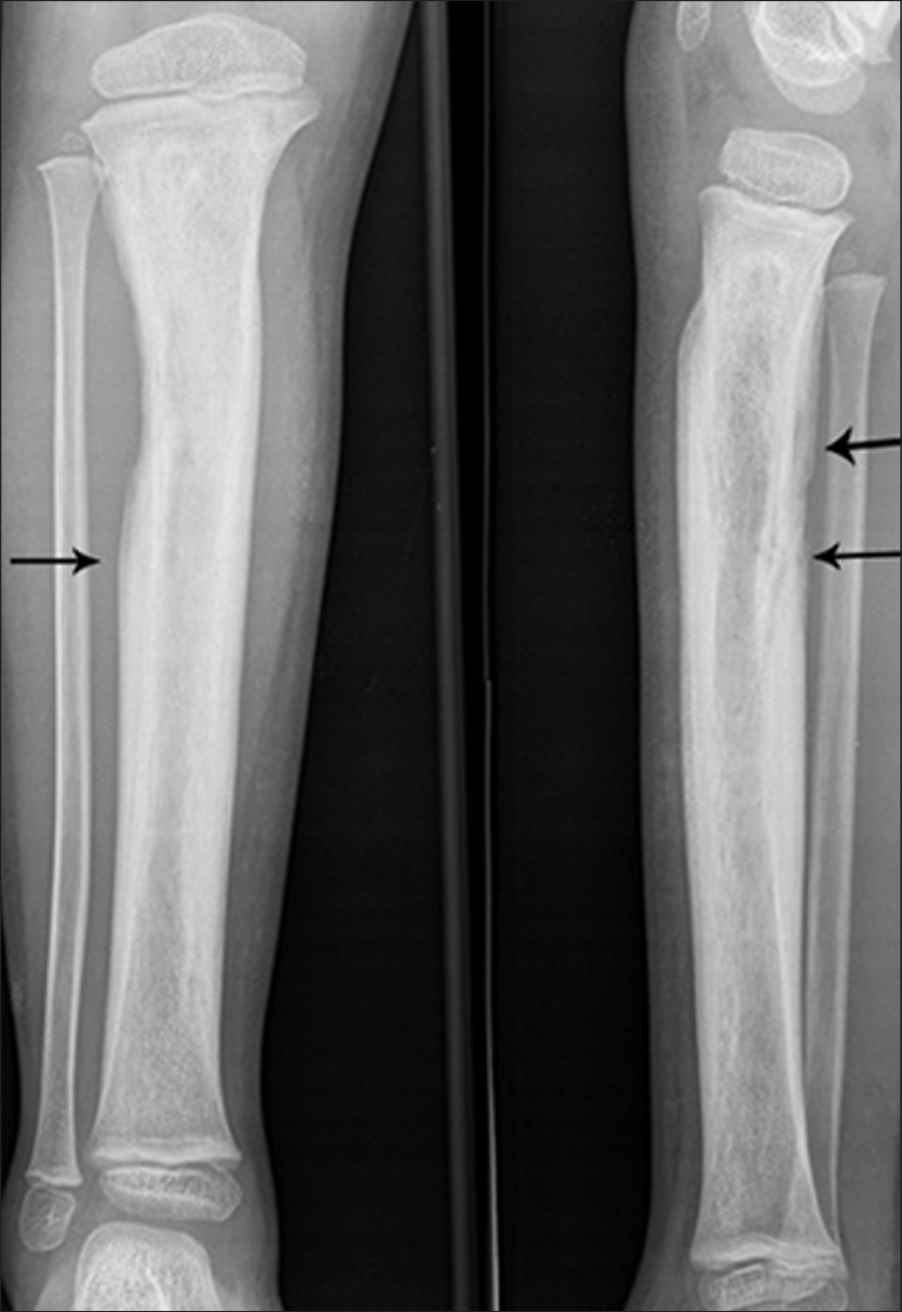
Extensive involucrum on plain radiography. Anteroposterior and lateral plain radiography showing extensive involucrum (arrow) at the tibial diaphysis (black arrows).
